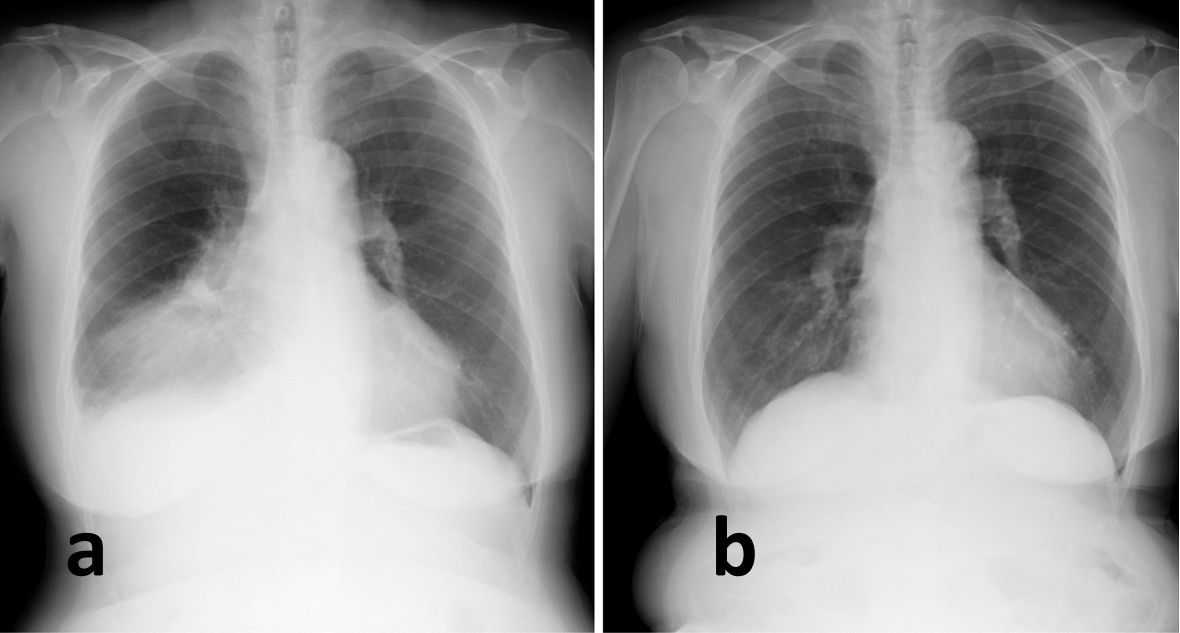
A chest radiograph on admission revealed a mass (a) which was not evident 6 months prior (b)
